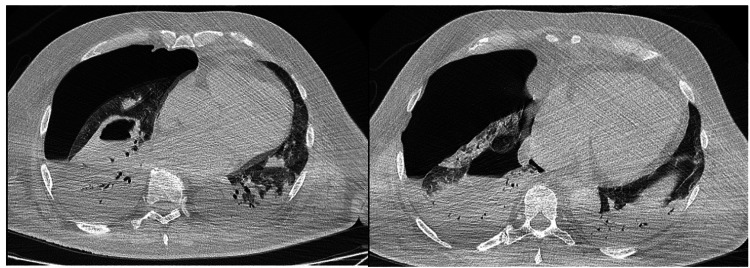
Chest computed tomography scan of a 63-year-old male with severe SARS-CoV-2 pneumonia and spontaneous right hydropneumothorax, with no previous chronic pulmonary disease.
